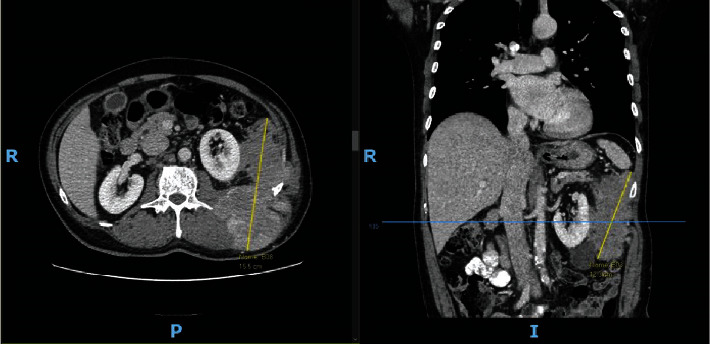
Preoperative CT scan of the patient. Note: CT scan showing an ill-defined mass in the left retroperitoneum and paracolic gutter, extending beyond the left posterior abdominal wall.
